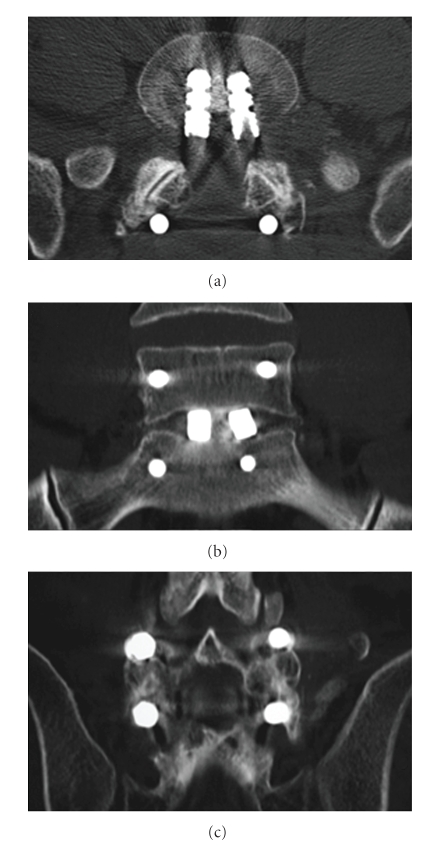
Computed tomography 6 months after surgery demonstrating solid L5-S1 circumferential fusion. (a) Axial section at the lumbosacral junction. (b) Coronal section at the middle portion of the L5 vertebral body. (c) Coronal section at the L5-S1 posterior fusion.
